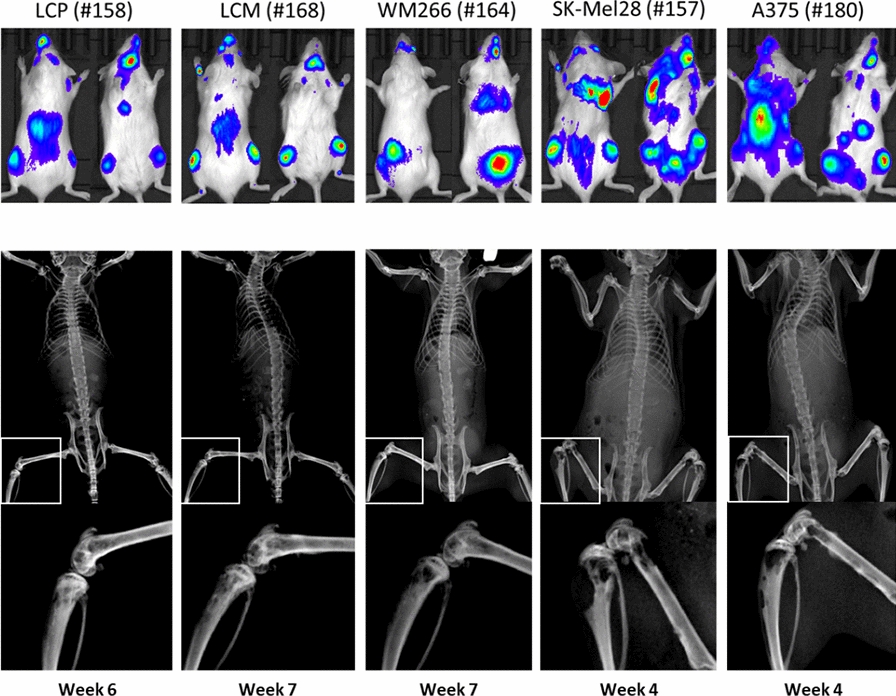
(Abstract 13) The CTA-AT cell line shows typical melanoma phenotype (a), an intermediate EMT phenotype (b–d) and molecules associated with immune escape (c)
